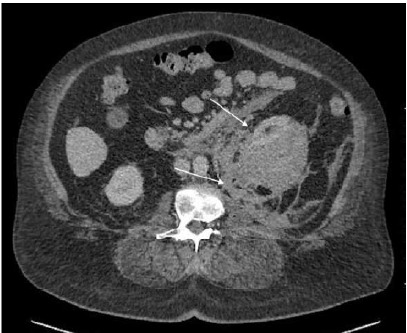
Computed tomography angiogram of the chest, abdomen, and pelvis, axial view, showing subcapsular renal hematoma of the left kidney with active extravasation (white arrows).
